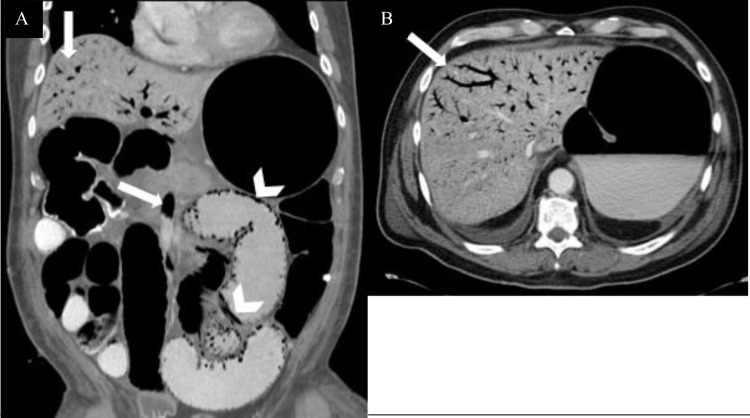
58-year-old patient status post multi-visceral transplant of liver, pancreas, and bowel with worsening abdominal pain after feeding trial. Coronal CT image A shows gas in the superior mesenteric and portal veins (arrows) and PI of several dilated small bowel loops (arrowheads). Axial CT image B shows branching gas in the liver periphery (centrifugal flow from portal vein toward periphery). Laparoscopic evaluation revealed extensive bowel ischemia and approximately 120 cm of small bowel was resected
